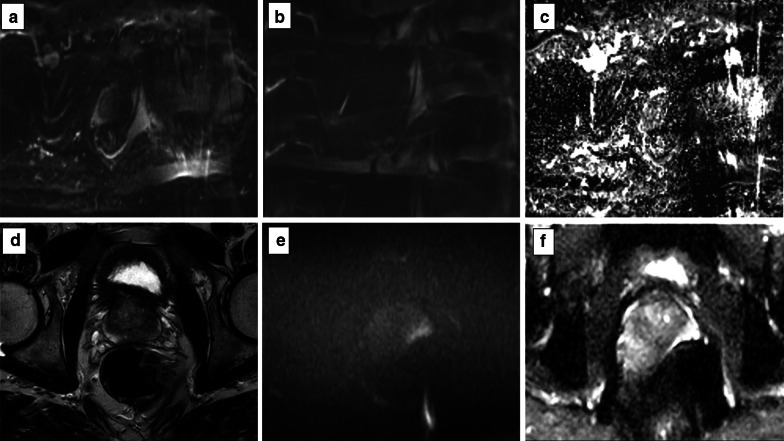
DWI hampered by artefacts from metallic implants (left hip) (*b* = 150 and 1400 s/mm^2^ in a, b, respectively, and ADC map in c) and rectal air (T2w imaging) in d showing the distended rectum, *b* = 1400 s/mm^2^ and ADC map in e, f, respectively)
